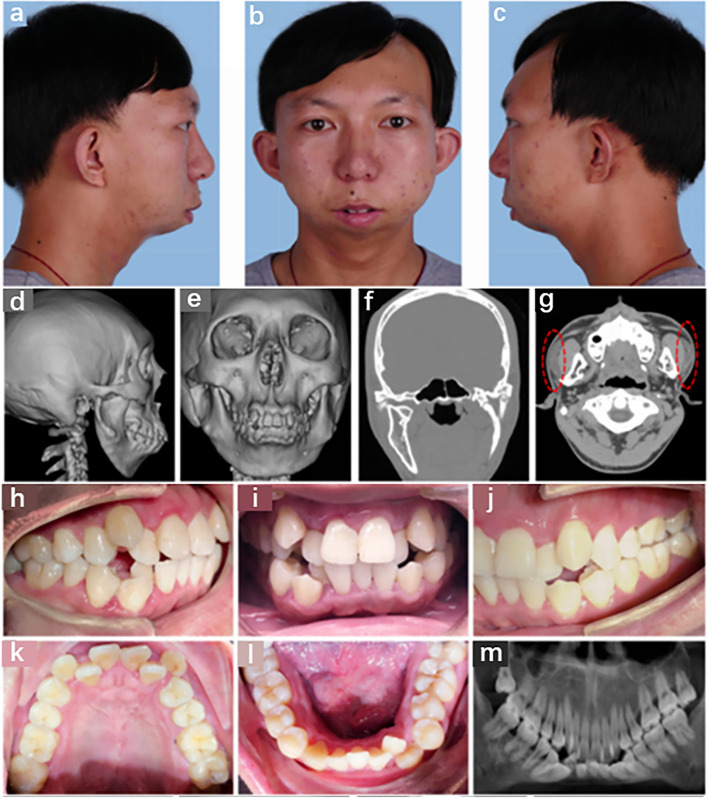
Phenotypic presentation of the patient’s younger brother. a-c The side view of the face showing similar micrognathia, full cheeks, and a round face shape. d-f Three-dimensional (3D) reconstruction of computed tomography (CT) data indicating micrognathia, bony ridge protruding on the mandibular rami, and mandibular condyle hypoplasia. g The red dashed lines indicate a heterotopic parotid gland. h–l Intraoral photographs showing the deep overbite and overjet of anterior teeth, disordered tooth and crowded dental arch. m The panoramic radiograph shows severe malocclusion
